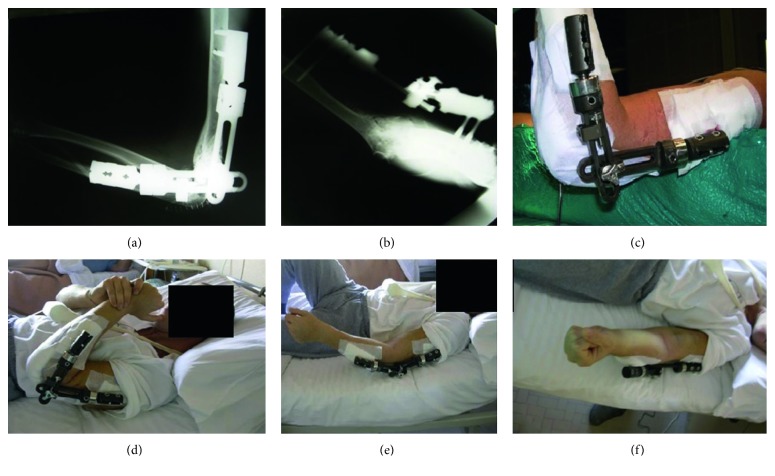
Postoperative situation. Postoperative radiographs after Grika interposition arthroplasty with hinged external fixator (a, b); active and passive motion during hospitalization (c–f).
